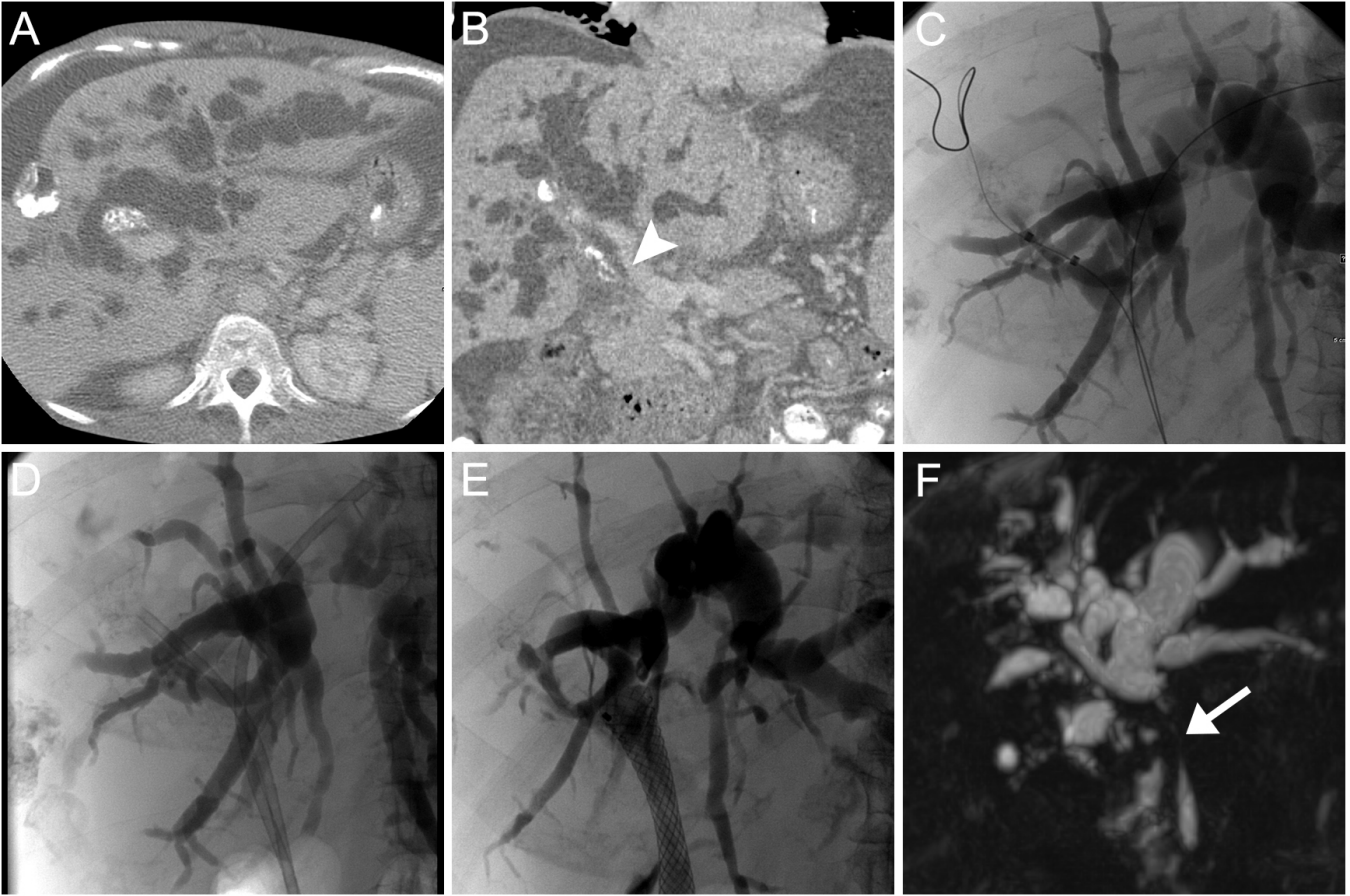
Imaging and ERC findings patient 3. CT scan in axial (A) and coronal (B) orientation and ERC (C) show bilateral obstructive cholestasis with dilatation of intrahepatic bile ducts as well as calcified mass lesions in the periphery and hilum of the liver. Infiltration of the liver hilum with stenosis of the main bile duct (arrow head). Balloon dilation of stenosis and insertion of plastic stents (D). Temporary placement of a FCSEMS (E). MRCP shows persistent stenosis of the main bile duct after extraction of FCSEMS (F) clinically there was”assisted clinical success” (see Fig 1).
